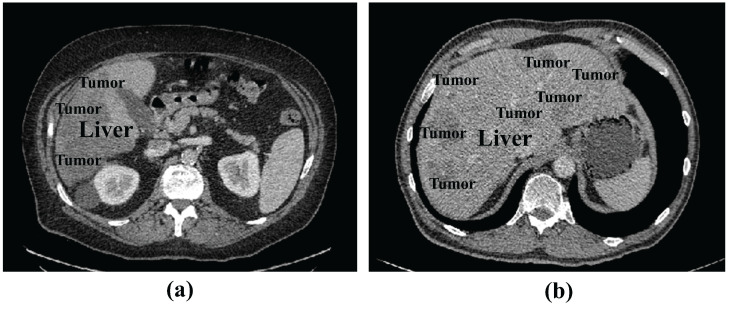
Examples of liver CT images: (a) tumors located around the liver boundary (b) small and low-contrast liver tumor.
